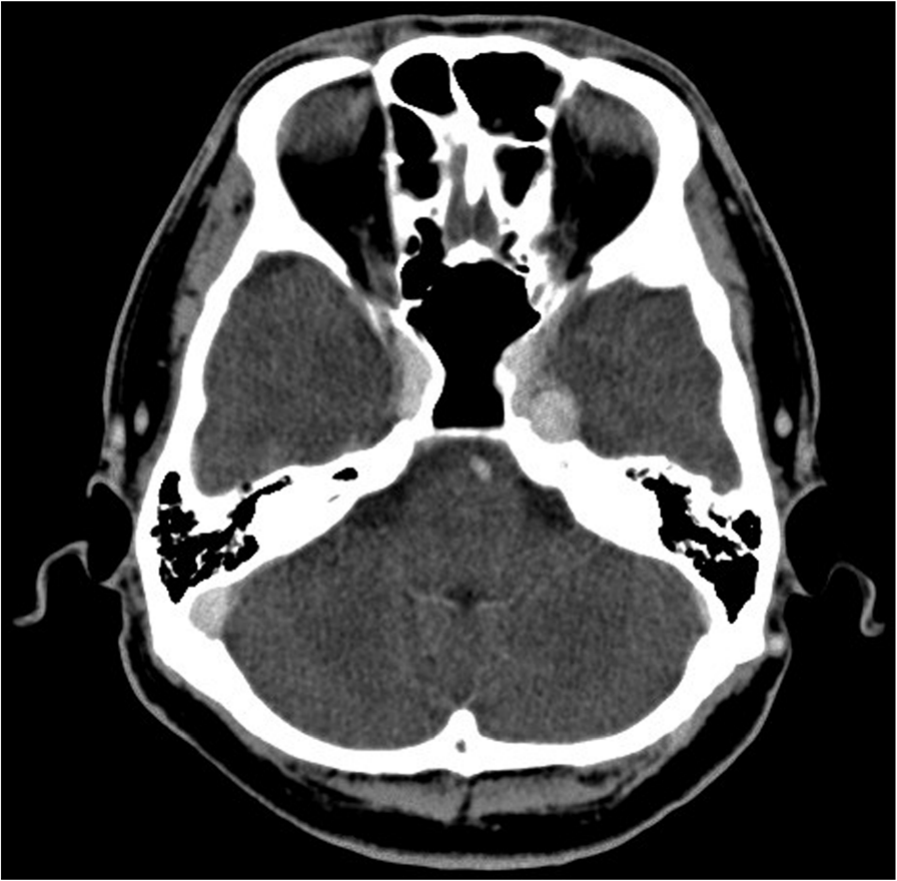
Unenhanced computed tomography image at referral hospital. A localized well-defined mass in Meckel’s cave, homogeneously hyperdense to the gray matter
